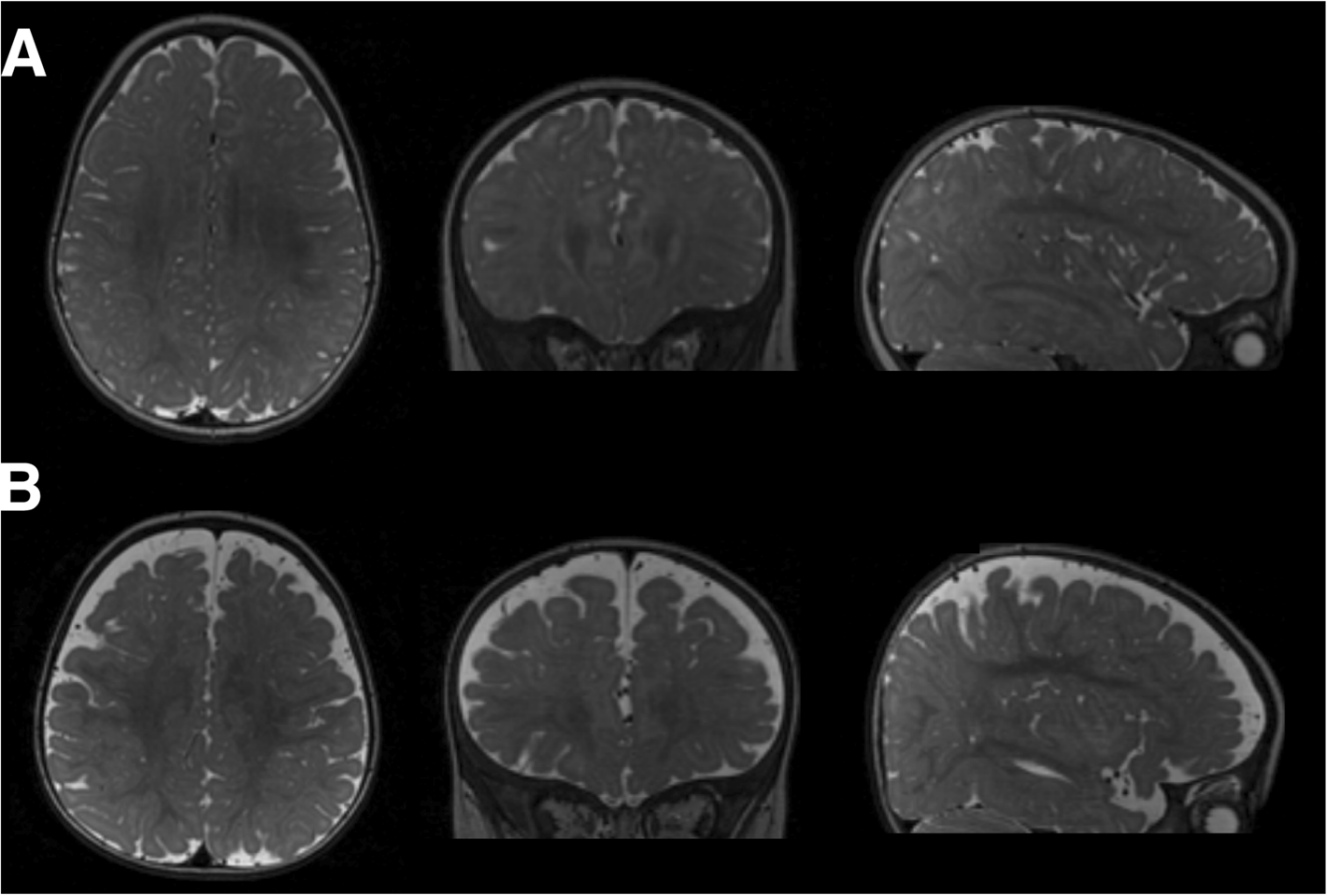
a T2-weighted images of an infant with a normal MRI at 6 months of age, who was confirmed as having typical development at 2 years of age. b Similar T2-weighted images of an infant with excessive extra-axial CSF at 6 months, who was diagnosed with ASD at 2 years of age. [CSF is indicated as brighter regions in these images. Images are of a horizontal section (left), coronal section (middle), and sagittal section (right) through the brain.]
